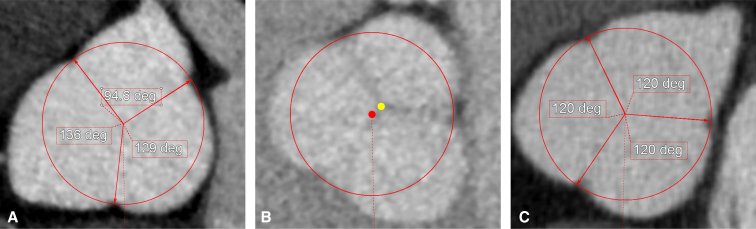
Computed tomography angiography imaging of aortic valves during preoperative assessment. Commissural alignment is often uneven (A). The coaptation point of the 3 cusps (B, *yellow*) may be displaced from the circle connecting the commissures (*red*). We prefer to preserve the native geometry rather than impose a symmetric 120°-120°-120° orientation (C).
